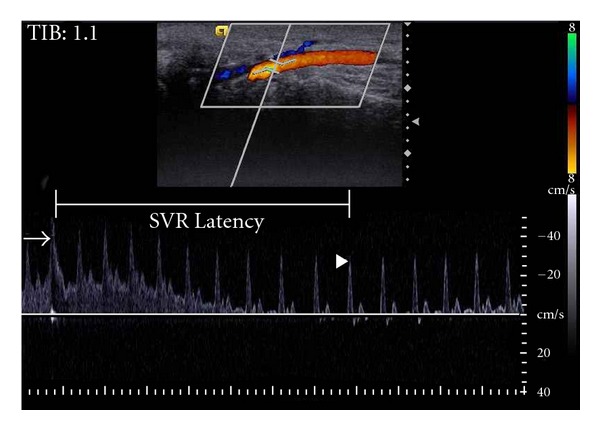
Change of Doppler wave forms upon sympathetic vasomotor response (SVR). The inspiratory cough causes an artifact (arrow) and decreases systolic blood velocity with a more prominent diastolic reversal. The wave of maximal deflection (arrowhead) was used to represent the hemodynamic status after sympathetic stimulation. The SVR latency indicated the period between the artifact by inspiratory cough to the maximal deflection of Doppler wave forms.
